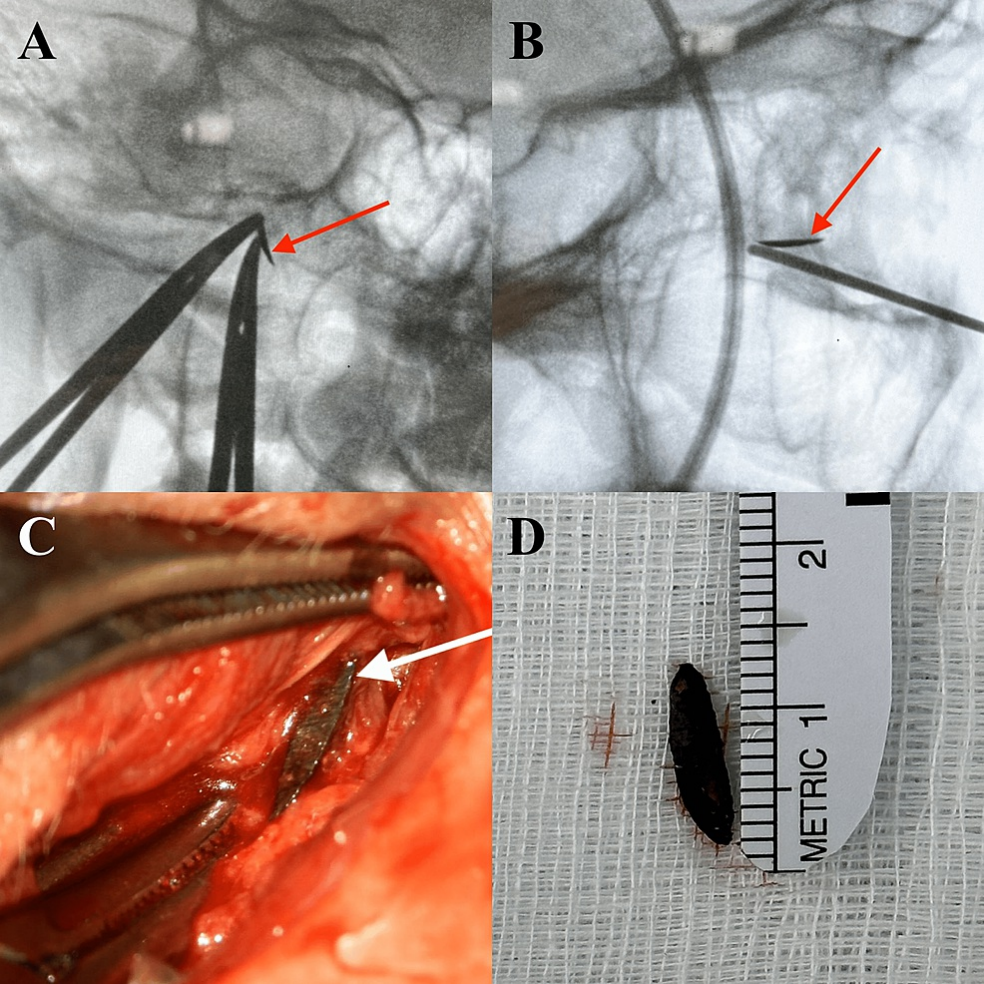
Radiographs and the IOFB IOFB: Intraorbital foreign body (A) Radiograph with mosquito forceps used as markers. (B) Radiograph with a blunt syringe needle used as marker. (C) Mosquito forceps (markers) intraoperatively and the foreign body (white arrow). (D) The foreign body
